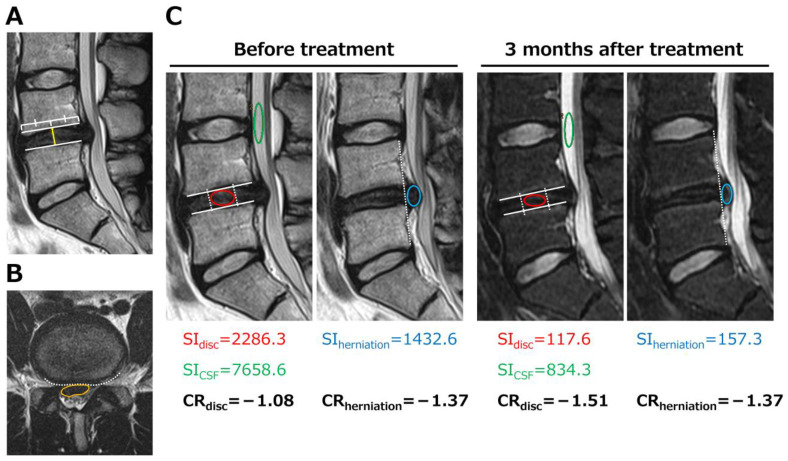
MRI findings. (A) Disc height at the midpoint of the vertebra (yellow line) on T2-weighted MRI. (B) The herniated mass area was quantified at the maximum bulging level on axial MRI (orange area), defined by reference lines drawn from the original dorsal surface of the annulus fibrosus (dotted line) to the dorsal surface of the herniated mass. (C) Images of the same patient before and after treatment. SI_disc_ was measured within the central 1/3 of the intervertebral disc (red area). SI_herniation_ was measured within the herniated region protruding beyond the posterior vertebral margin (dotted line) (blue area). SI_csf_ was obtained from the cerebrospinal fluid at the lumbar lesion level (green area). The contrast ratios (CR) were calculated as follows: CR_disc_ = (SI_disc_ − SI_csf_)/((SI_disc_ + SI_csf_)/2), and CR_herniation_ = (SI_herniation_ − SI_csf_)/((SI_herniation_ + SI_csf_)/2).
